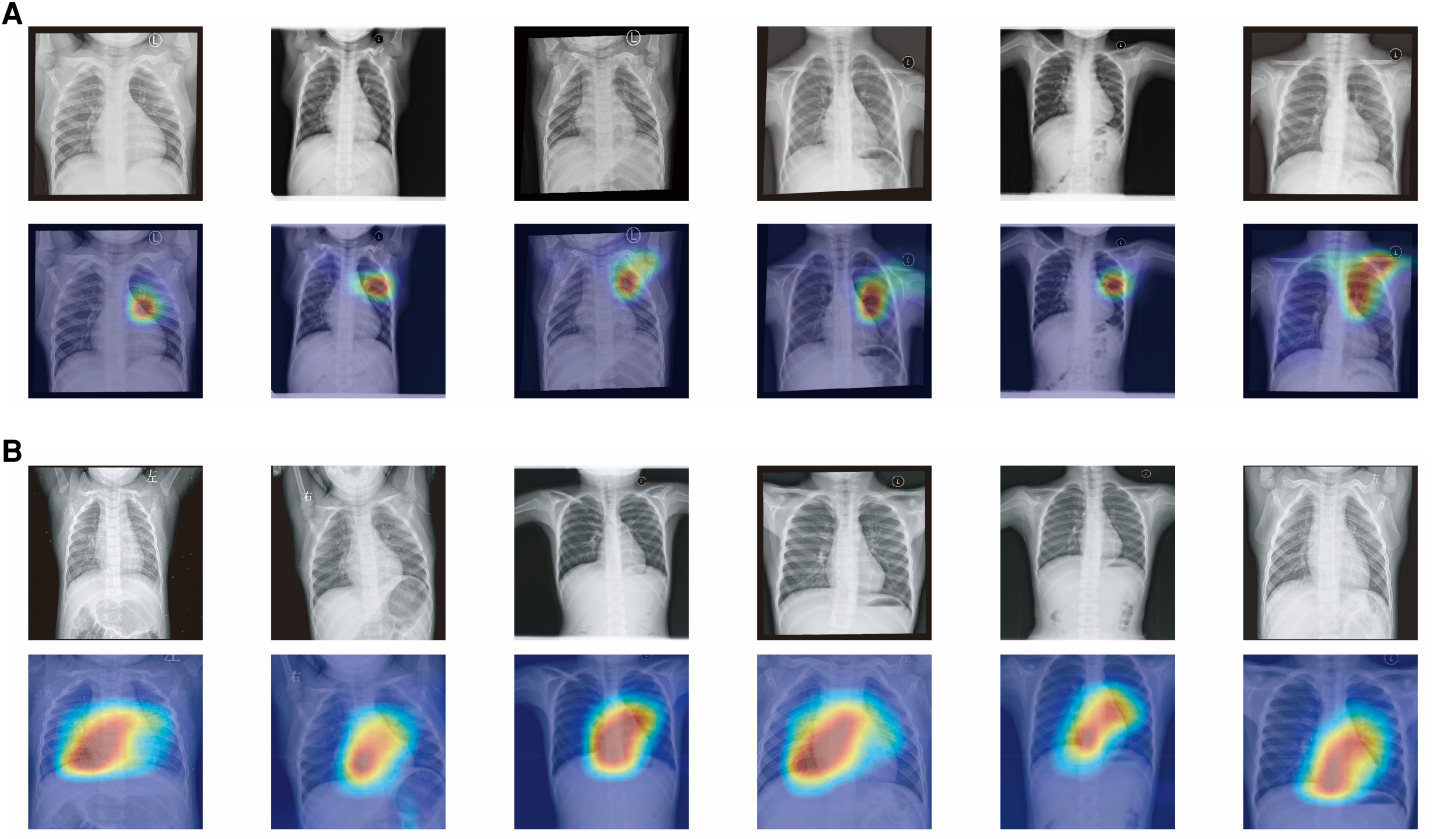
(A) Attention map of chest x-ray model for nonPAH-VSD patients. (B) Attention map of chest x-ray model for PAH-VSD patients.
